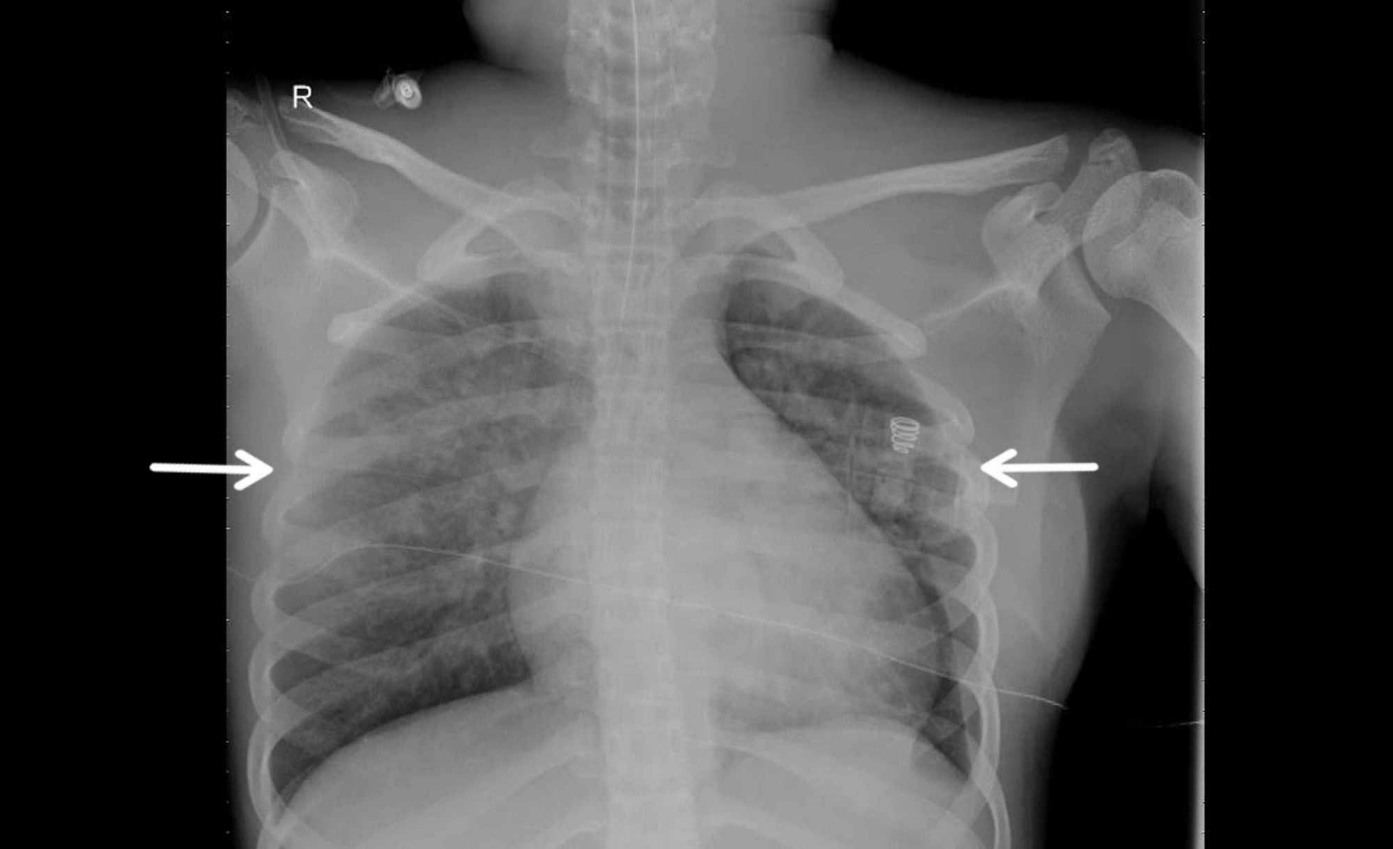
The patient’s chest X-ray is showing acute whiteout of the lung bilaterally consistent with acute respiratory distress syndrome. The X-ray is also showing that the patient is intubated.
